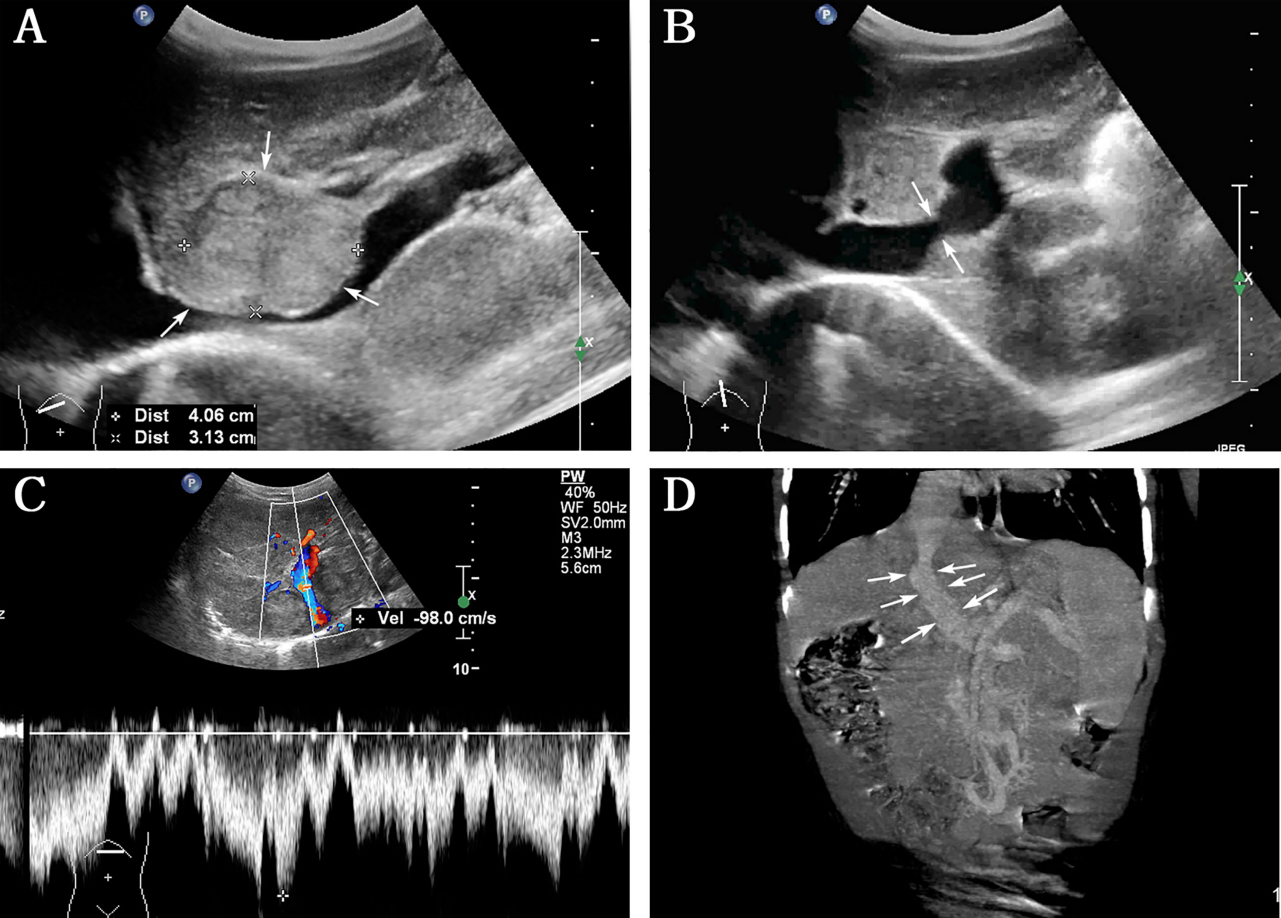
Abdominal ultrasound and contrast-enhanced computed tomography (CT) were performed before interventional therapy. (A) Grayscale indicates the largest hepatic nodule (arrows) with clear boundary and inferior vena cava (IVC) compression; (B) left portal vein directly communicated with IVC (arrows); (C) color Doppler ultrasound image confirmed PV flow toward IVC, with a flow velocity of 98 cm/s in the shunt; (D) contrast-enhanced CT showed the presence of direct communication between PV and IVC (arrows).
